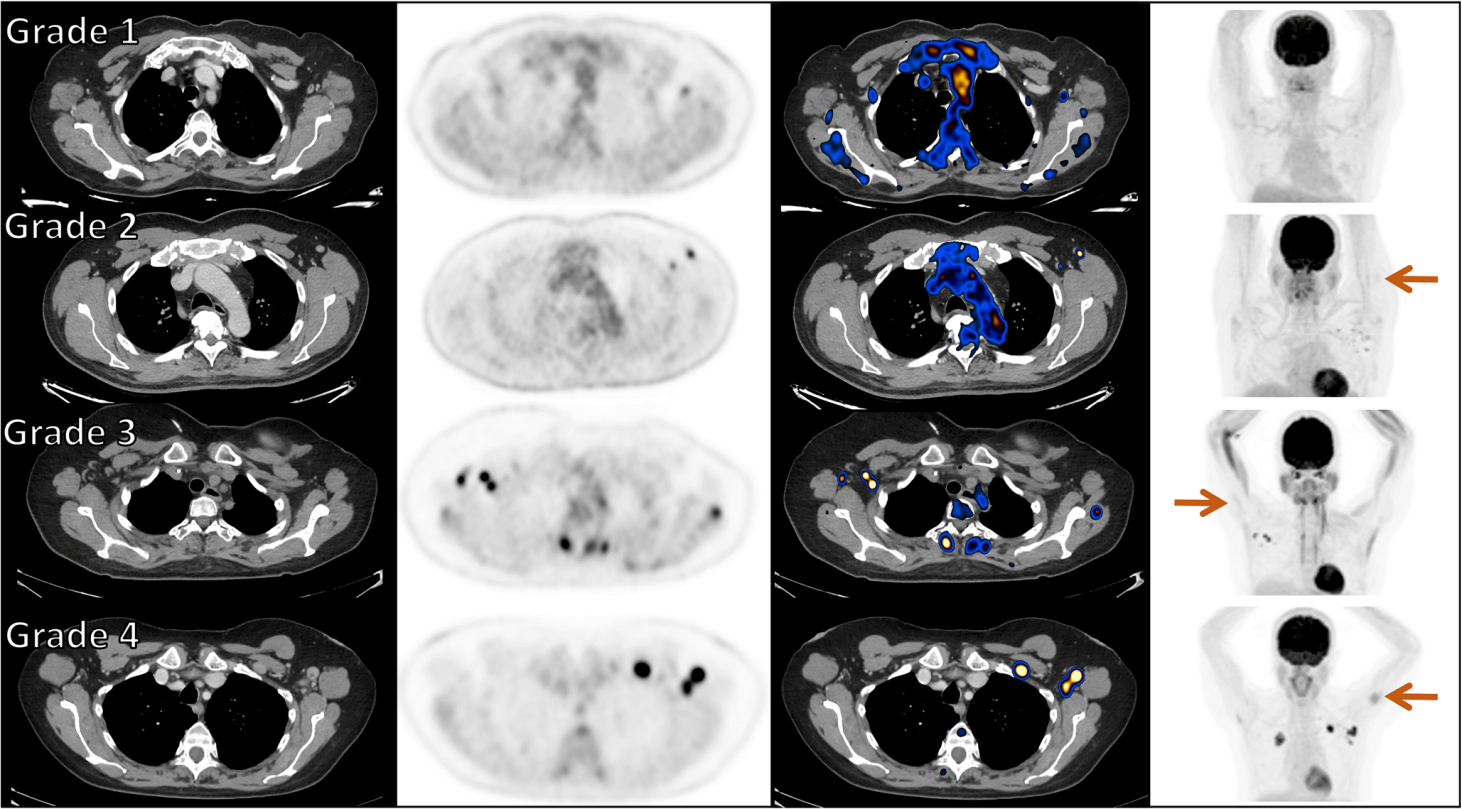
Vaccine-associated hypermetabolic lymphadenopathy (VAHL) grades. Each row represents one patient and includes from left to right CT, PET, and fusion PET-CT trans-axial slices and a maximal intensity projection (MIP) image. From top to bottom: patient referred for staging of colon cancer imaged 9 days following the first vaccine dose, patient referred for follow-up of rectal cancer 13 days following the booster vaccine dose, patient with history of left breast cancer referred for follow-up study 10 days following the first vaccine dose, and a patient referred for staging of right upper lobe lung cancer 1 day following the booster vaccine dose. In all presented cases, HLN was identified in ASLN, attributed to the recent vaccination on the report, and graded in our data based on [^18^F]FDG-uptake intensity. SUVmax measured in the presented cases were 1.97, 3.39, 10.10, and 14.34 from top to bottom. On the bottom row, LN diameter was 14 mm. On the MIP images, brown arrows point hypermetabolism recognized at the vaccine injection site
